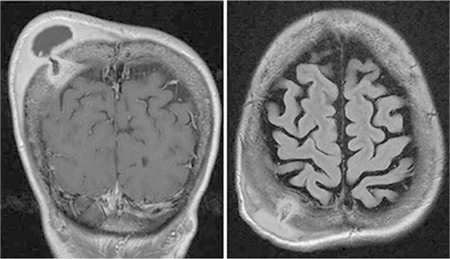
Magnetic resonance imaging of the brain demonstrating peripheral enhancement of the scalp abscess alongside noticeable enhancement of the adjacent soft tissues, pathological bone, and underlying pachymeningeal layer enhanced on a coronal T1W slice (left) with a clear intra-osseous edematous edema in keeping with osteomyelitic changes isolating a central bone sequestrum, communicating with a subperiosteal/subgaleal fluid collection on a fluid attenuated inversion recovery weighted slice (right).
